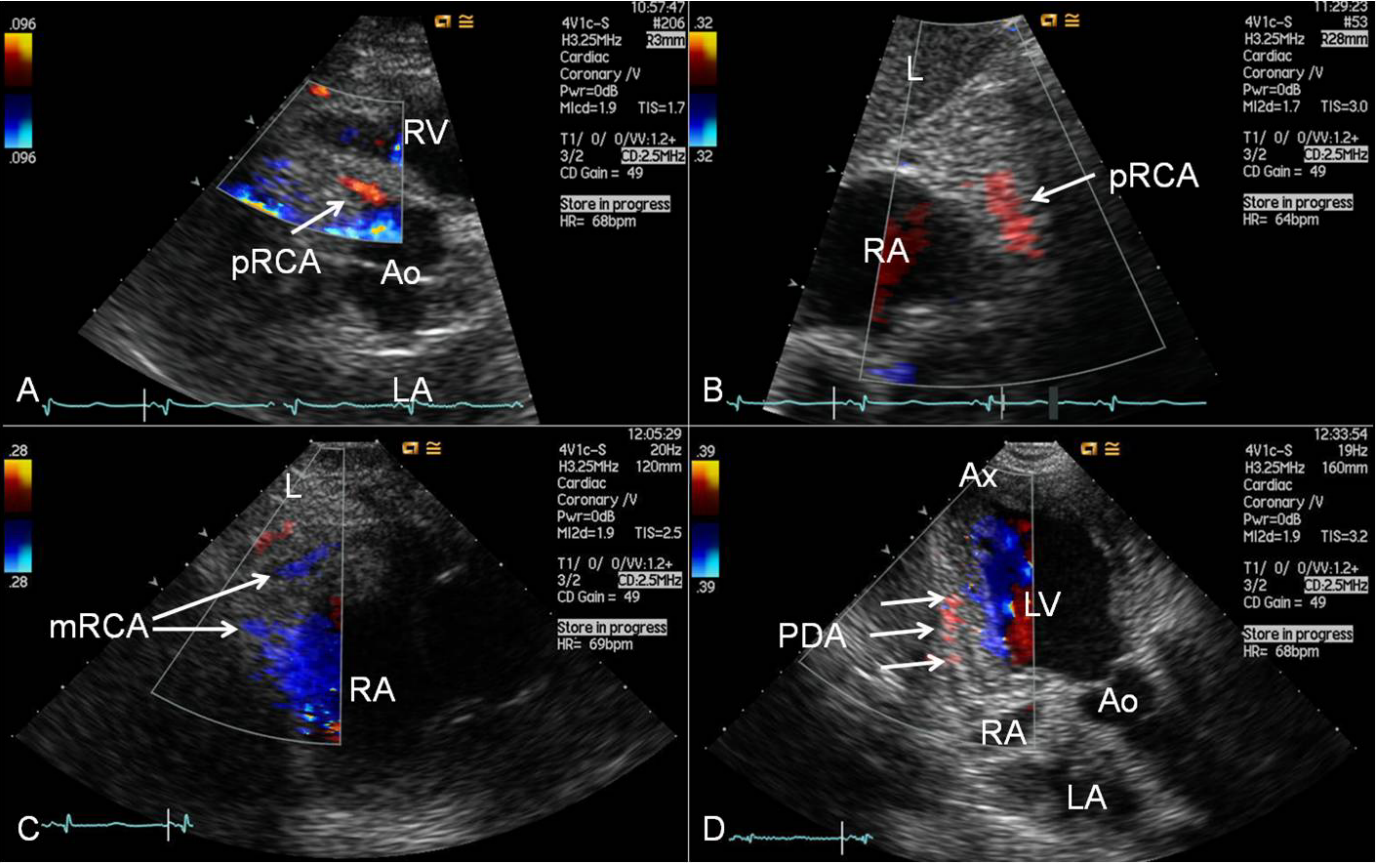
Examples of antegrade coronary artery flow in the RCA and PDA. (A) In modified parasternal short-axis view searching the area adjacent to the right sinus of Valsalva the proximal right coronary artery (pRCA) is seen leaving the aortic root (Ao). (B) From subcostal modified sagittal view the pRCA is seen traversing caudally on the anterior tricuspid ring. (C) Using subcostal modified short-axis view parts of the middle segment of the right coronary artery (mRCA) are seen coursing medially on the medial tricuspid ring. (D) From modified apical 2-chamber view focusing on the posterior interventricular sulcus parts of the posterior descending coronary artery (PDA) are seen coursing toward the apex (Ax). L = liver; LA = left atrium; LV = left ventricle; RA = right atrium; RV = right ventricle.
